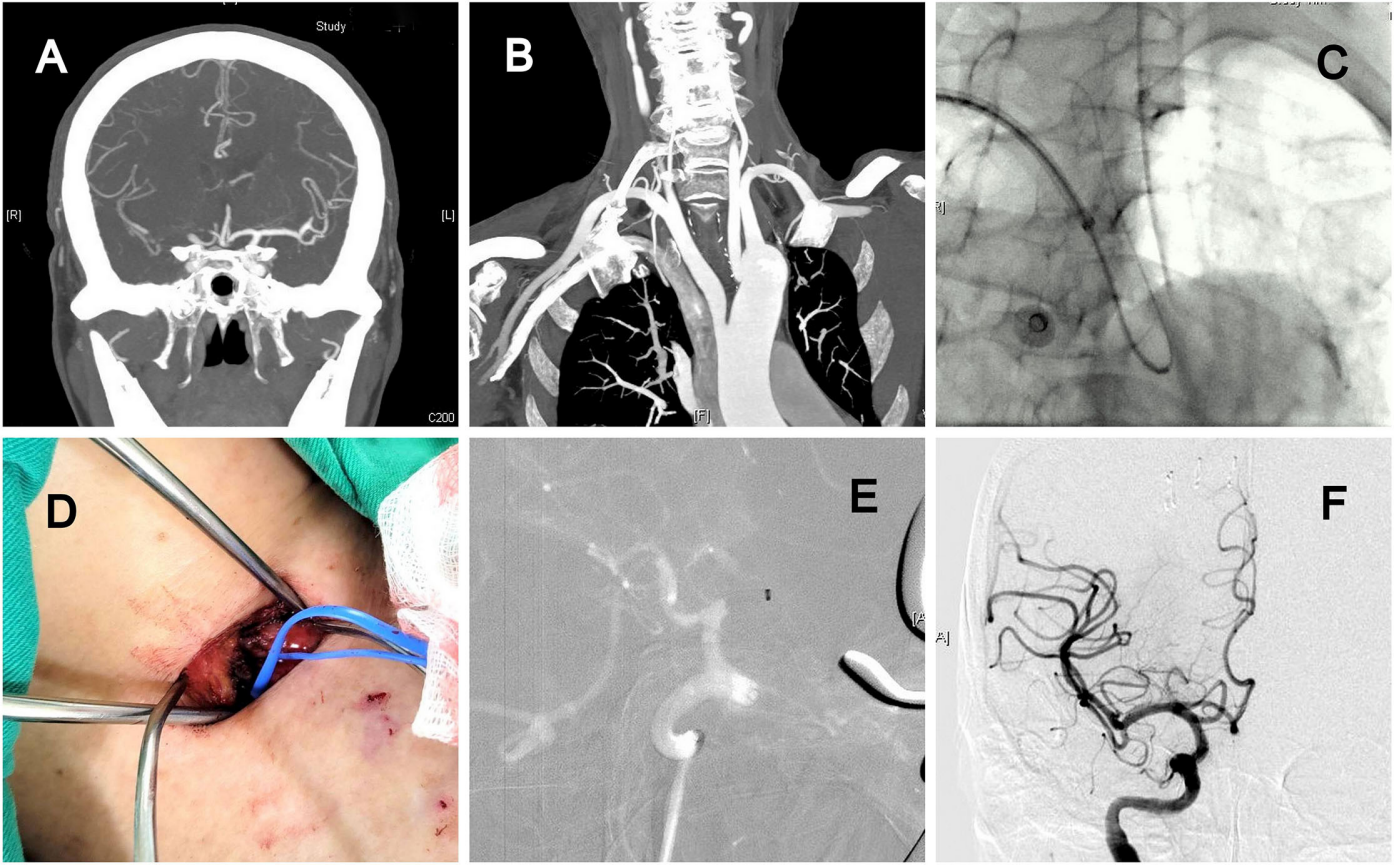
A patient had acute right middle cerebral artery (MCA) occlusion with a type III aortic arch and sharp angle between the right subclavian artery and right common carotid artery (CCA). (A) CT angiography (CTA) indicating total occlusion of the right MCA. (B) Type III aortic arch. (C) Sharp angle, which hindered the advancement of the long sheath to the carotid artery from the right hand. (D) Carotid artery exposure through a 2-cm skin incision. (E) Direct aspiration for mechanical thrombectomy. (F) Complete recanalization of the MCA.
